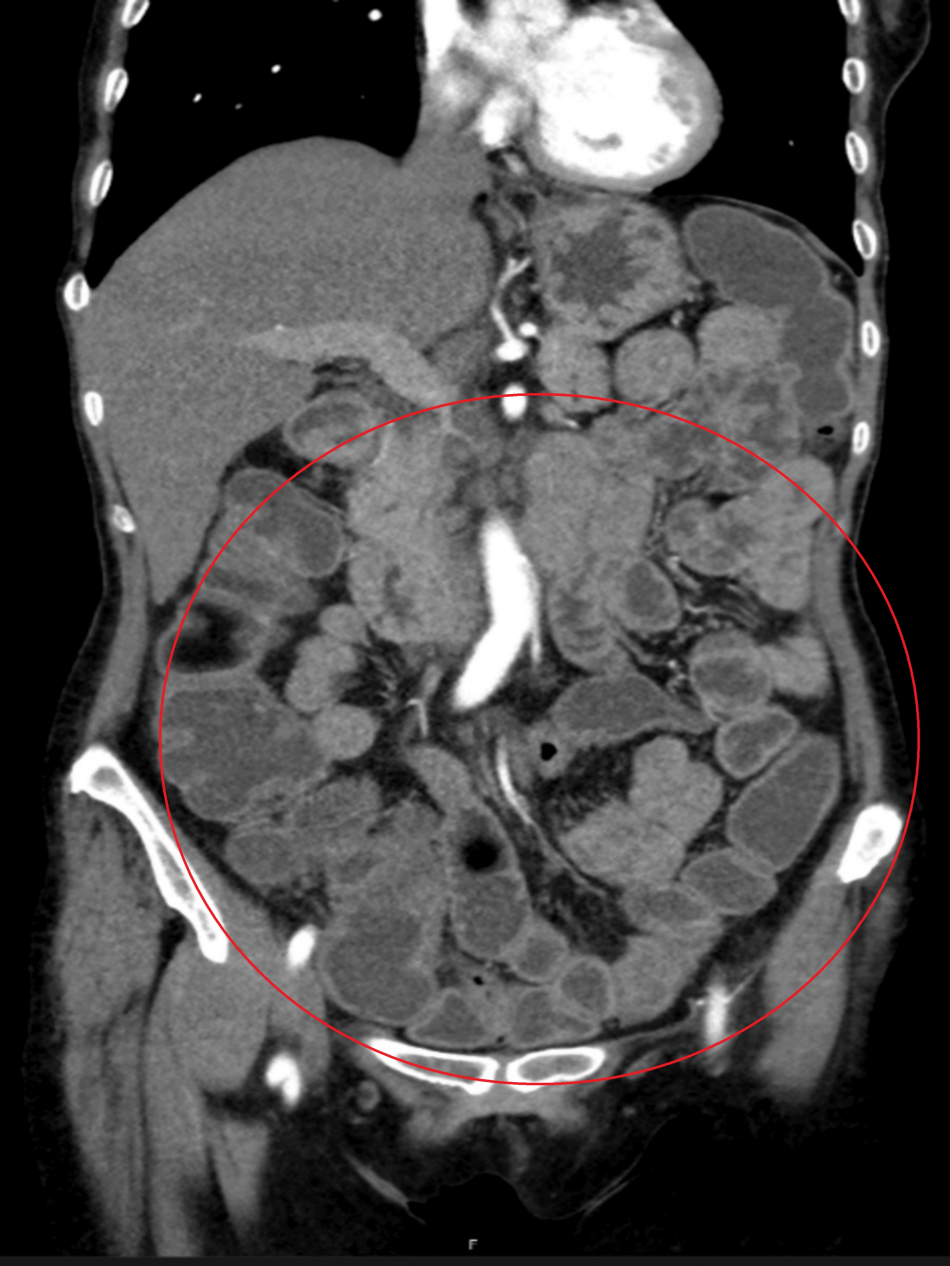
Abdominal CT scan: semi-distended small bowel loops with increased wall thickness, predominantly in the left hypochondrium and flank regions (red circle), at the beginning of the disease.
